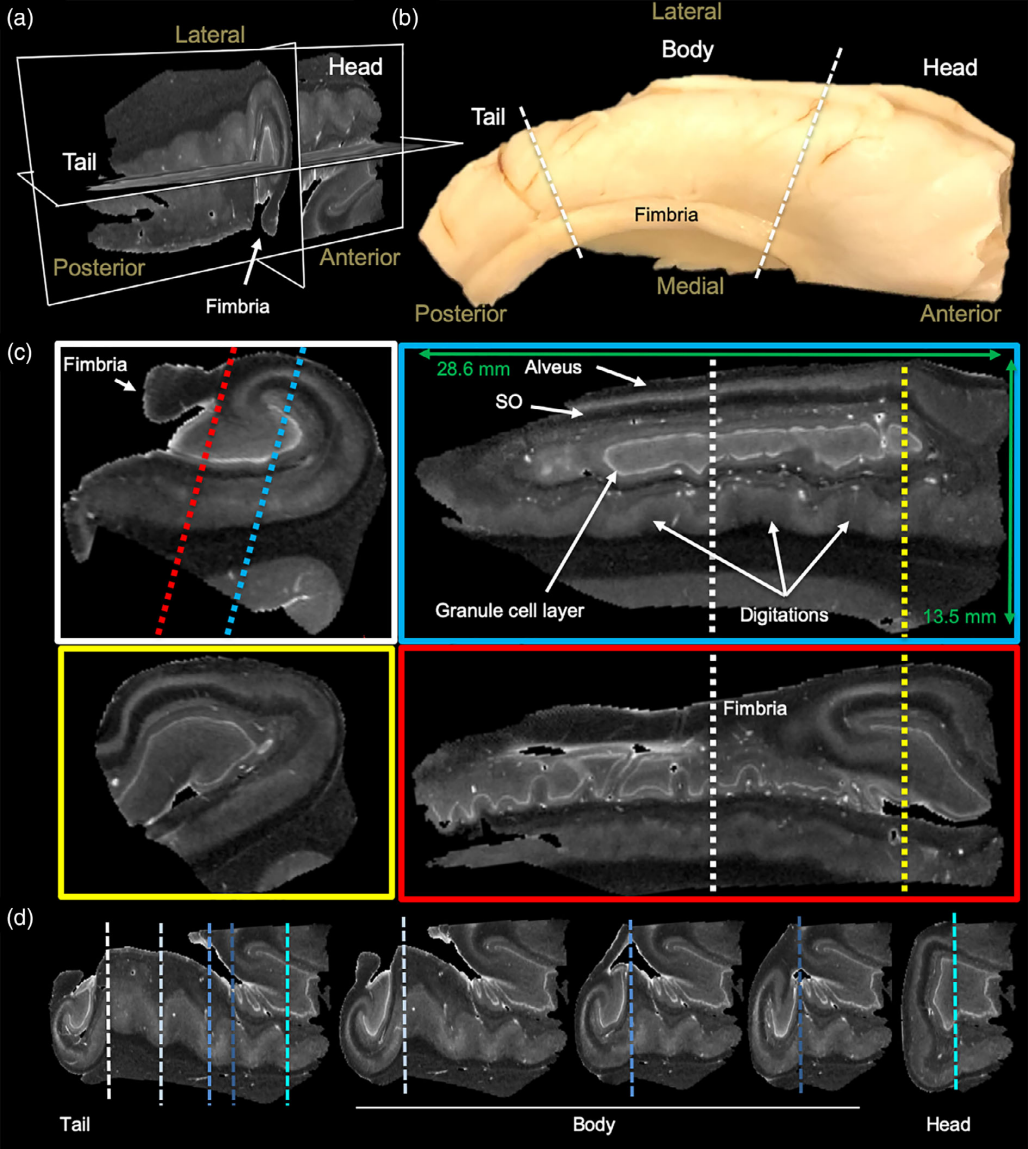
High resolution MR imaging of a whole healthy human hippocampus. (a) Visualization of the three axes of a 100 μm isotropic mean diffusion (MD) image. (b) Photograph of excised hippocampal sample and delineation of the tail, body and head regions. (c) Coronal and saggital sections of the hippocampus reveal signal differences between cell layers with the granule cell layer being more hyperintense than other regions. The stratum oriens (SO) and alveus are easily discernible in the saggital plane. (d) Anterior–posterior comparison of coronal slices
